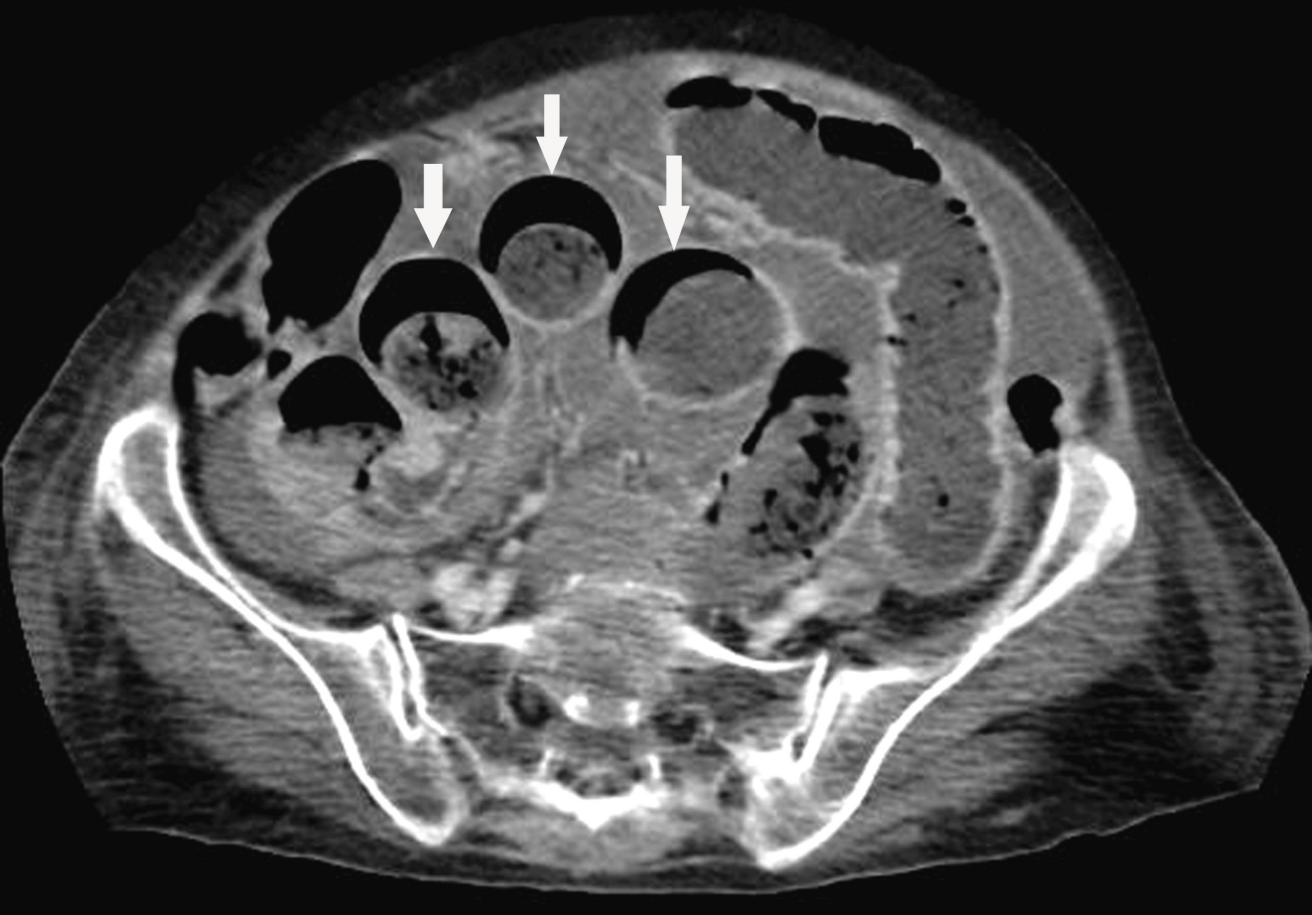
Axial contrast-enhanced CT scan of the abdomen. Showing significant curved-shaped accumulation of gas in the wall of the jejunum (arrows).
